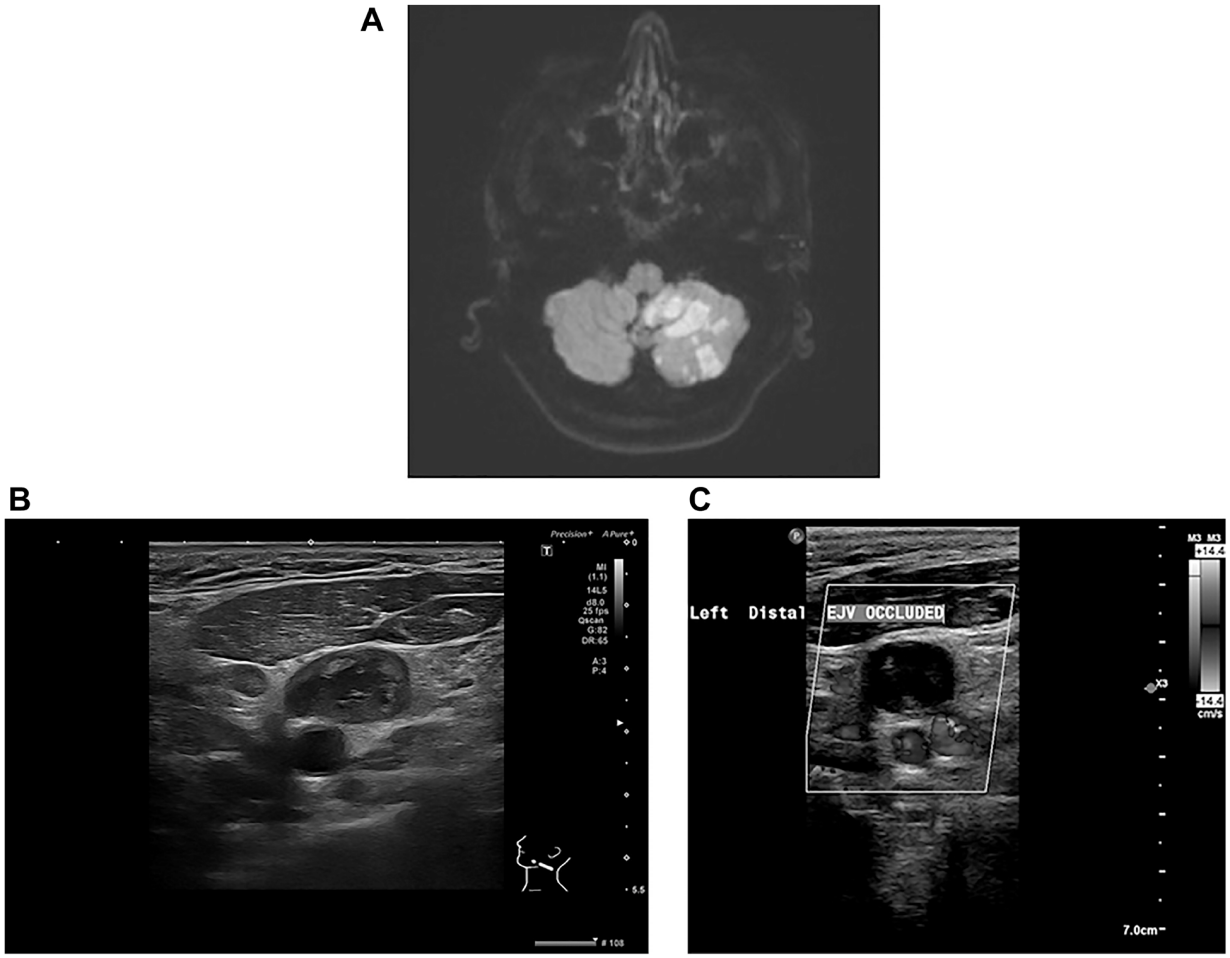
Multi-modality imaging findings in a patient with Trousseau’s syndrome and metastatic colorectal cancer. (A) Diffusion-weighted MRI of the brain demonstrating multifocal acute infarcts in multiple vascular territories, consistent with an embolic event. (B) Ultrasound of the neck revealing an enlarged supraclavicular lymph node with internal heterogeneity, raising suspicion for metastatic involvement. (C) Doppler ultrasound of the left neck demonstrating complete occlusion of the external jugular vein (EJV), consistent with malignancy-associated thrombosis.
